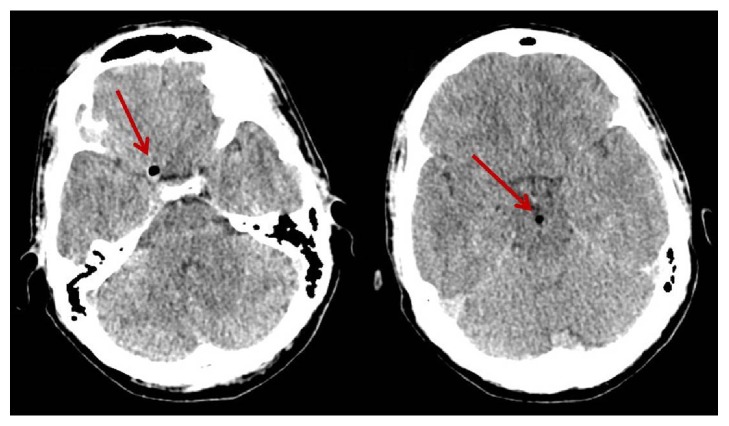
Cranial CT scan showing pneumocephalus.
